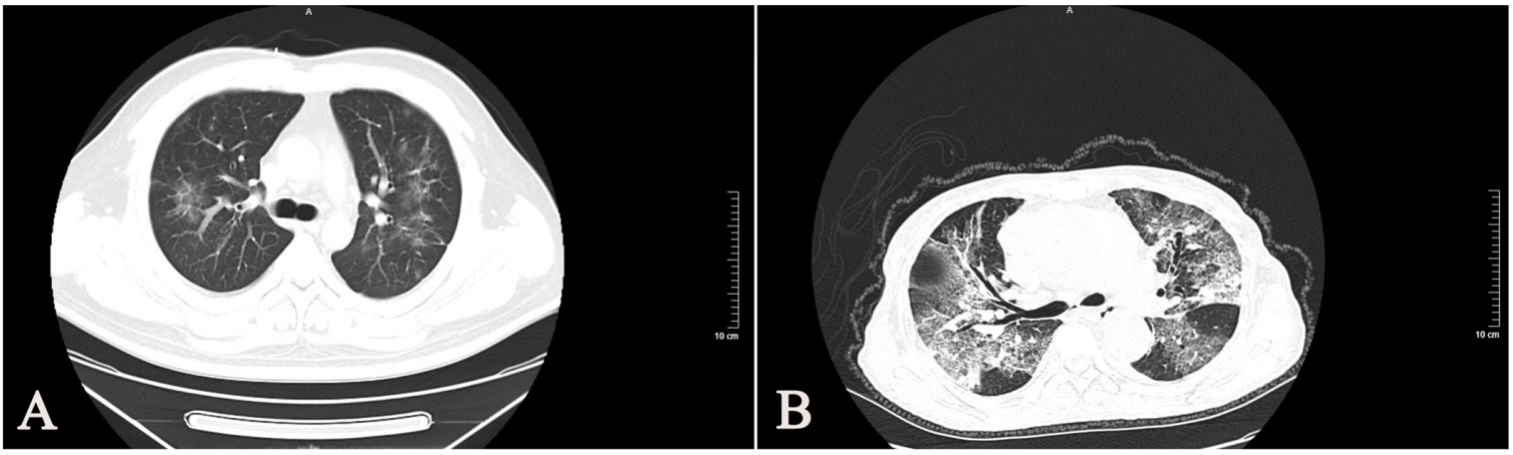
Chest CT images of SARS-CoV-2 infected patients. (A) A38-year-old man with confirmed SARS-CoV-2, moderate type. CT images show bilateral diffuse ground-glass opacities. (B) A 66-year-old woman with confirmed SARS-CoV-2, critically ill type. CT images show bilateral diffuse ground-glass opacities and reticulation.
